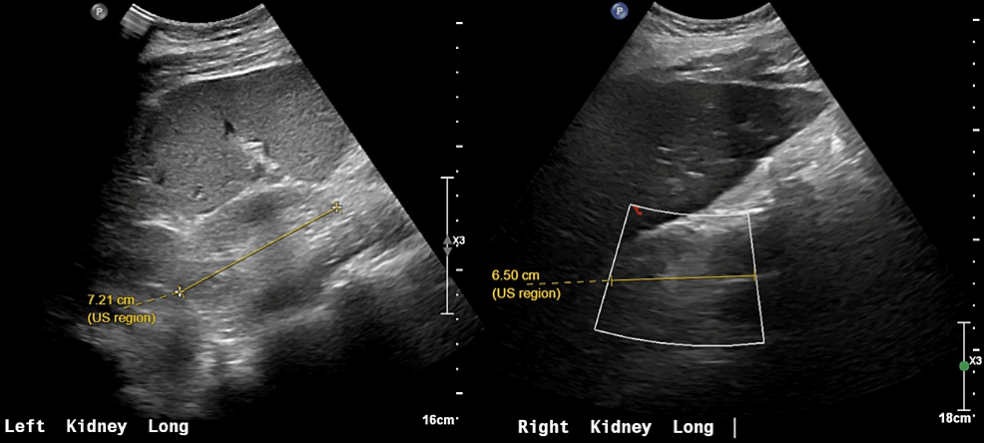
Renal ultrasound The left kidney (left) measured 7.2 cm. The right kidney (right) measured 6.5 cm, although the inferior margin was noted to be obscured by bowel gas.
